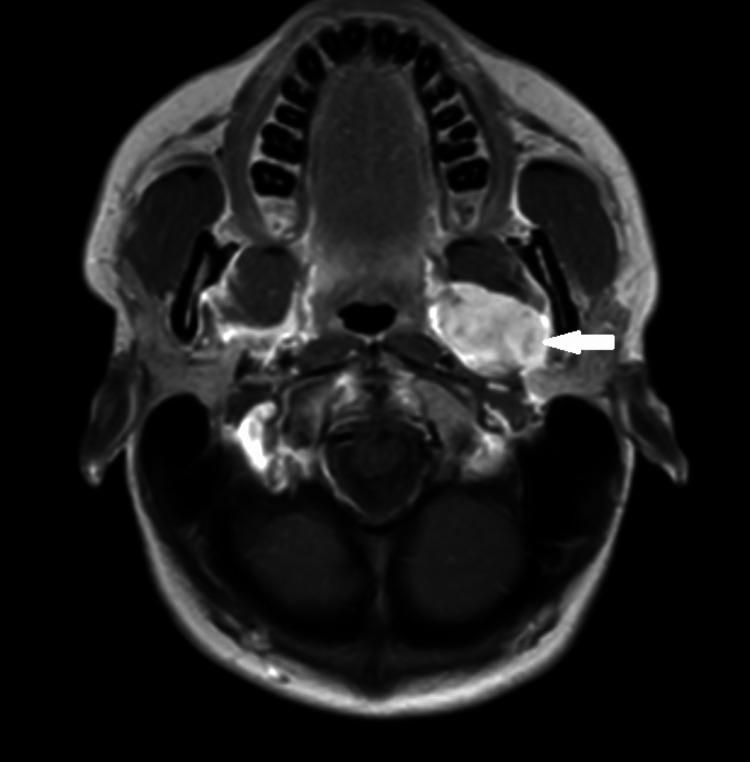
MRI (T1 transverse) showing the tumor in the parapharyngeal space (white arrow)
